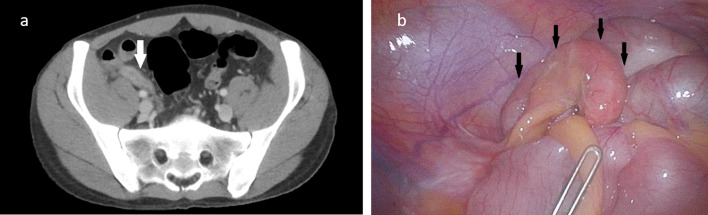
Radiological and laparoscopic features of acute appendicitis in a patient with neutropenia. Abdominal computed tomography showed appendicitis with phlegmonous inflammatory reaction surrounding the appendix without abscess or appendicolith (a, white arrow). Laparoscopically, the appendix vermiformis appears phlegmonously altered, but not purulent (b, black arrows)
